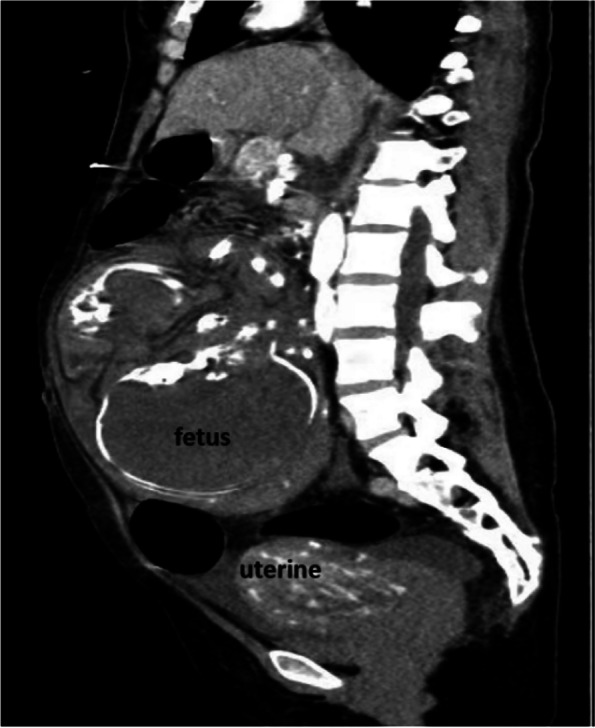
Abdominal CT revealing an empty uterus and a longitudinal fetus outside
